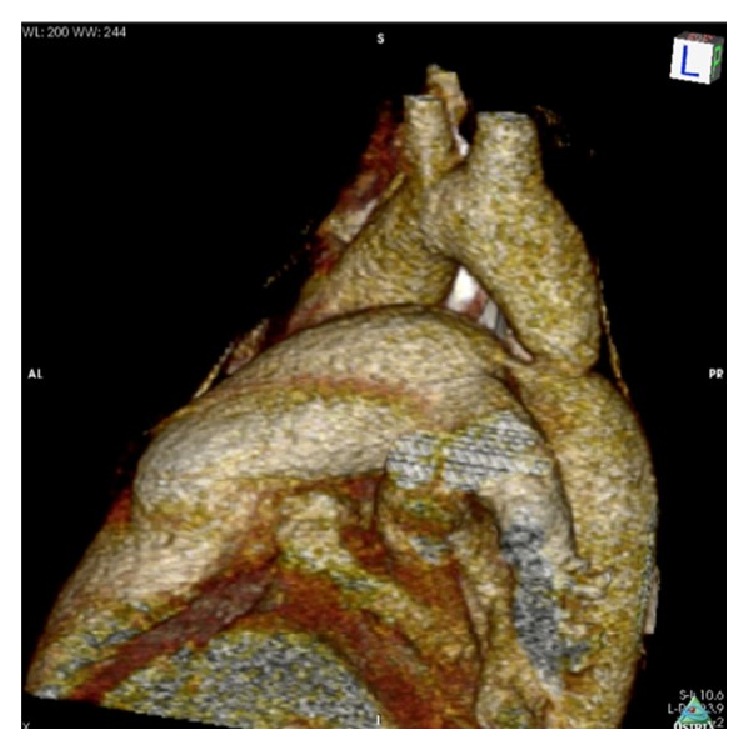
Thorax CT angiography, a strict aortic coarctation proximal part of descending aorta, and a small size patent ductus arteriosus were detected.
